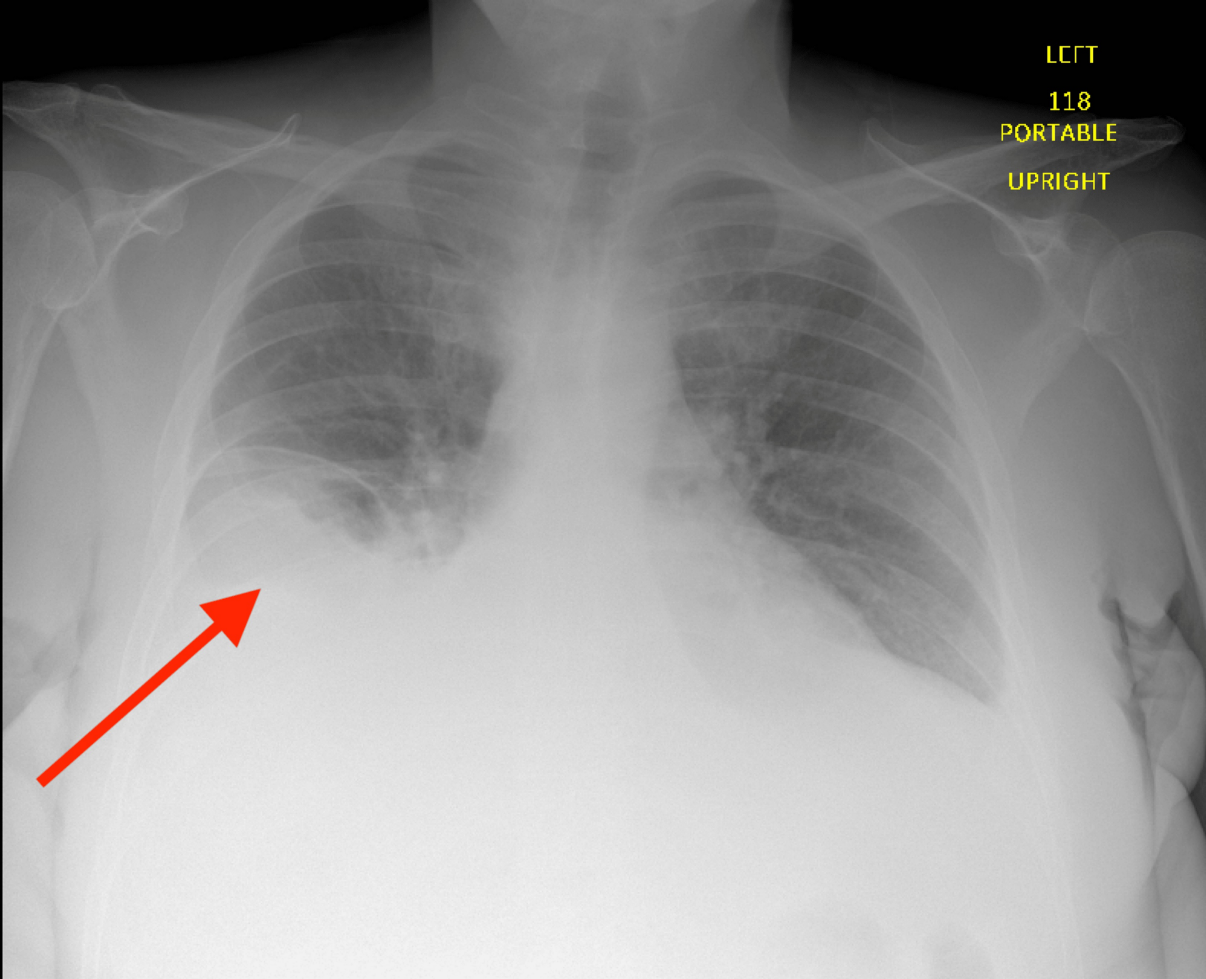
Chest radiograph showing bilateral pleural effusions, right greater than left (arrow)
